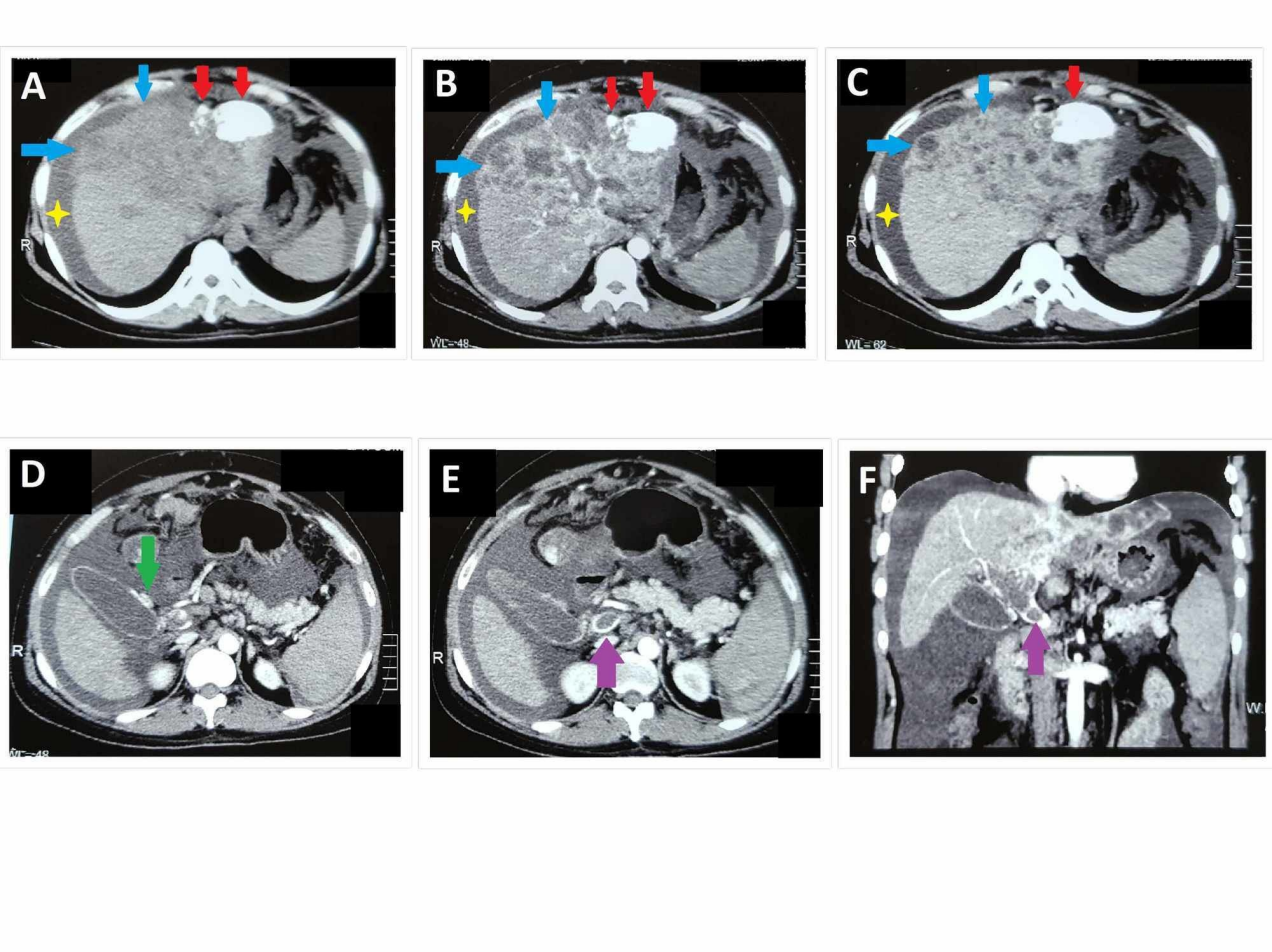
Two months post-TACE triphasic CT abdomen showing plain (A), arterial (B), and venous (C) phases of the study Two areas of dense hyper-densities (red arrows) are identified in the left lobe of liver-post TACE changes. There are newly developed multiple ill-defined infiltrative lesions (blue arrows) involving the left and right lobes of liver showing arterial hyperenhancement (B) and washout on venous phases (C). The liver has irregular margins and nodular contour, and there are moderate abdominopelvic ascites (yellow star). Dilated vascular channels are noted within the wall of the gallbladder (D) representing the varices formation (green arrow). These are showing arterial filling suggestive of AV shunting due to primary hepatic malignancy. Portal vein (purple arrow) is dilated showing arterial hyperenhancement along with a filling defect, suggestive of AV shunting and portal vein thrombosis. Peripancreatic lymph nodes are also seen. CT: computed tomography; TACE: transarterial chemoembolization; AV shunting: arteriovenous shunting
